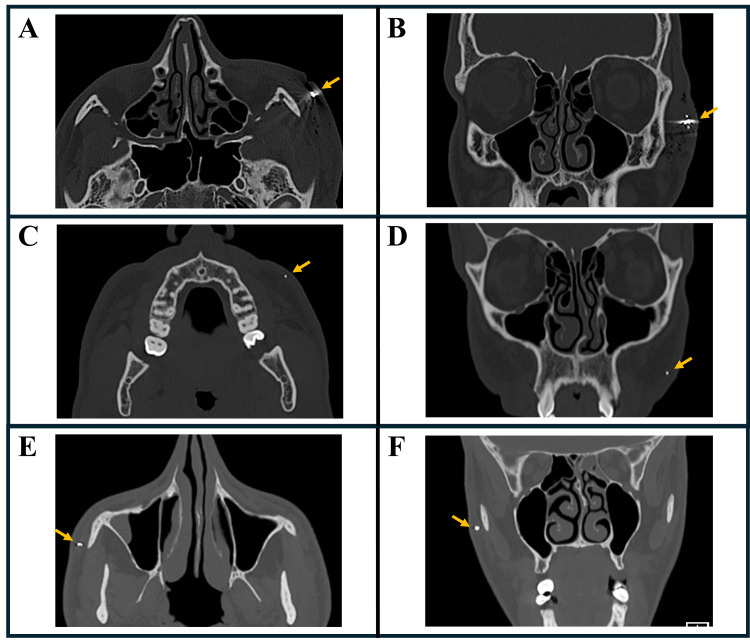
CT imaging of metallic foreign bodies in cases 2-4. CT images showing the precise locations of metallic foreign bodies (yellow arrows) in the facial regions of cases 2-4. (A, B) Case 2: Axial and coronal slices reveal three metallic fragments in the left zygomatic area. (C, D) Case 3: Axial and coronal views display a single metallic fragment in the left buccal region. (E, F) Case 4: Axial and coronal sections highlight a metallic fragment in the right zygomatic area.
